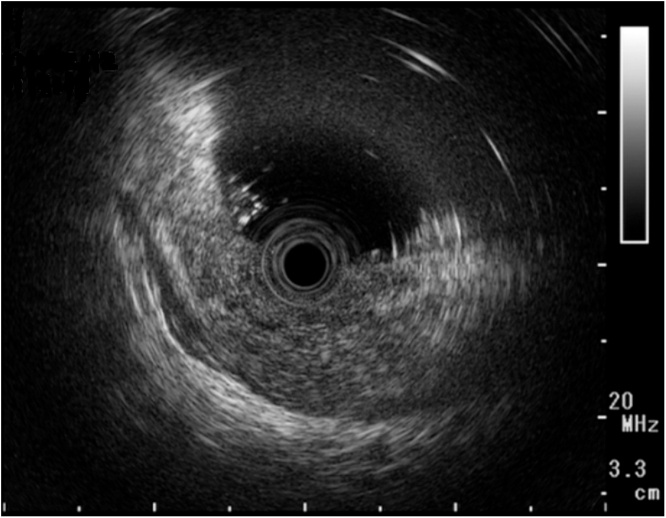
Endoscopic ultrasonography showed a low echoic tumor extending into the fourth layer of the gastric wall.
